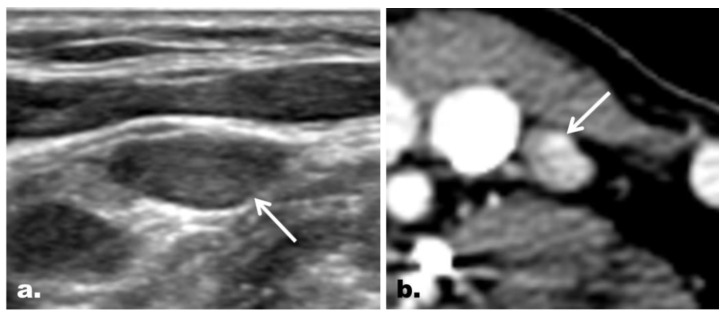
Representative case of correct reclassification of a US indeterminate LN with the addition of CT in a 48-year-old woman with PTC. (a) On US, an ovoid LN (arrow) with neither echogenic hilum nor suspicious feature was noted at the left neck level IV. The LN was classified as an indeterminate LN on US. (b) The LN, however, was shown to have a focal strong contrast enhancement (arrow) on CT, which led to correct reclassification of the LN as a suspicious LN. The final diagnosis based on FNA turned out to be metastasis. Correct changes in the classification were made in 5.1% (14 of 274) of the LNs enrolled, and the addition of CT helped identify additional metastasis in 6.2% (14 of 225) of the patients enrolled.
